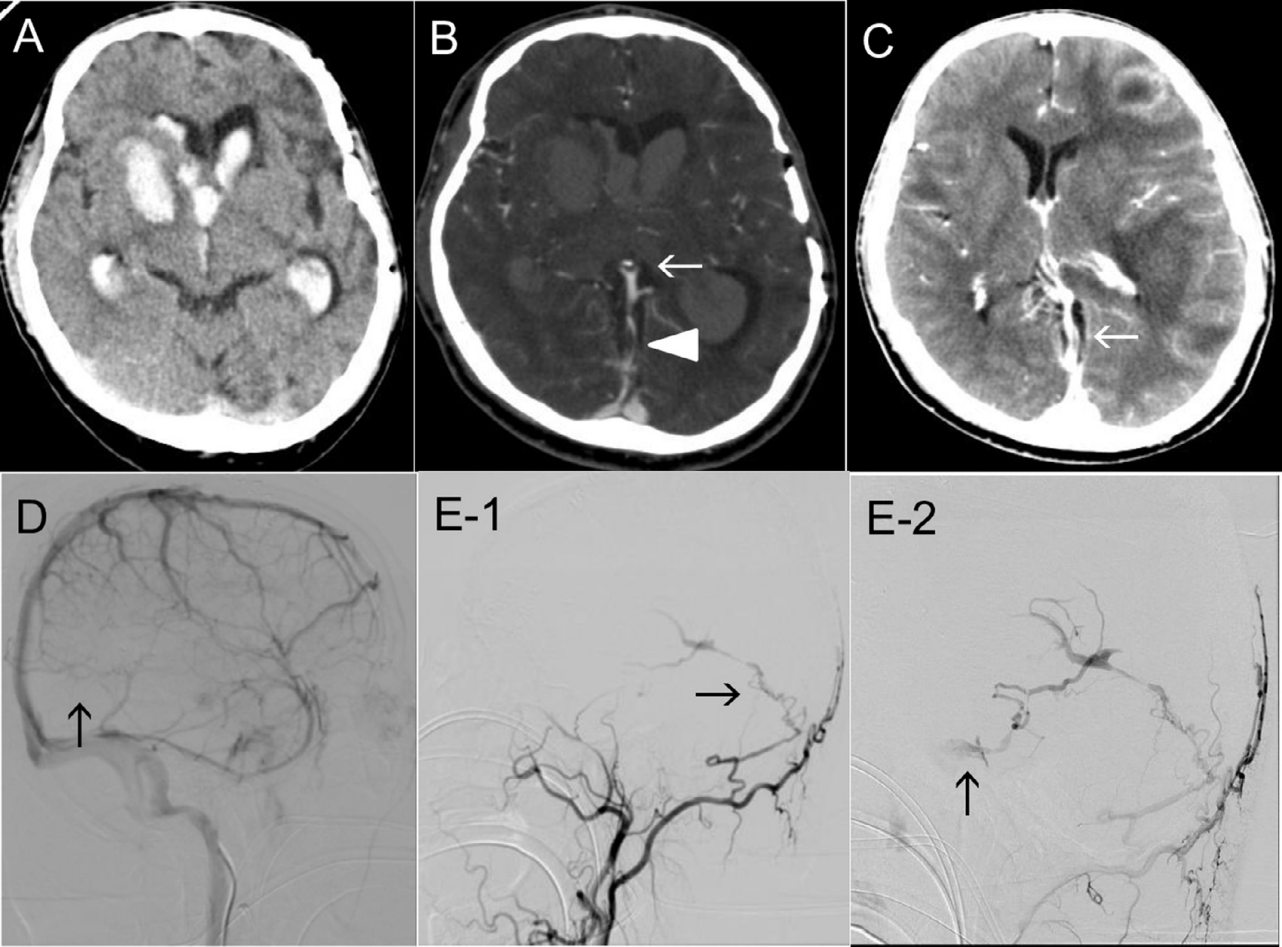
(A) Axial CT on admission showing a right putaminal hemorrhage and acute hydrocephalus due to an intraventricular hematoma. (B) Axial CT angiography image showing the bilateral basal vein and the straight sinus (StS) in the arterial phase (arrow), indicating an arteriovenous shunt. Additionally, contrast CT showing a partial defect of the StS (arrowhead), indicating sinus thrombosis. (C) Contrast-enhanced axial CT at the time of multiple brain abscesses 2 years earlier showing patency of the StS (arrow). (D) Venous phase of right ICAG showing StS occlusion (arrow). (E) Left ECAG showing DAVF shunt point from the mastoid branch of the OA to the StS (E-1, arrow). The venous reflux flows to the left cavernous sinus through the basal vein and uncal vein (E-2, arrow) (E-1, E-2: lateral view). CT, computed tomography; DAVF, dural arteriovenous fistula; ECAG, external carotid artery angiography; ICAG, internal carotid artery angiography; OA, occipital artery; StS, straight sinus.
